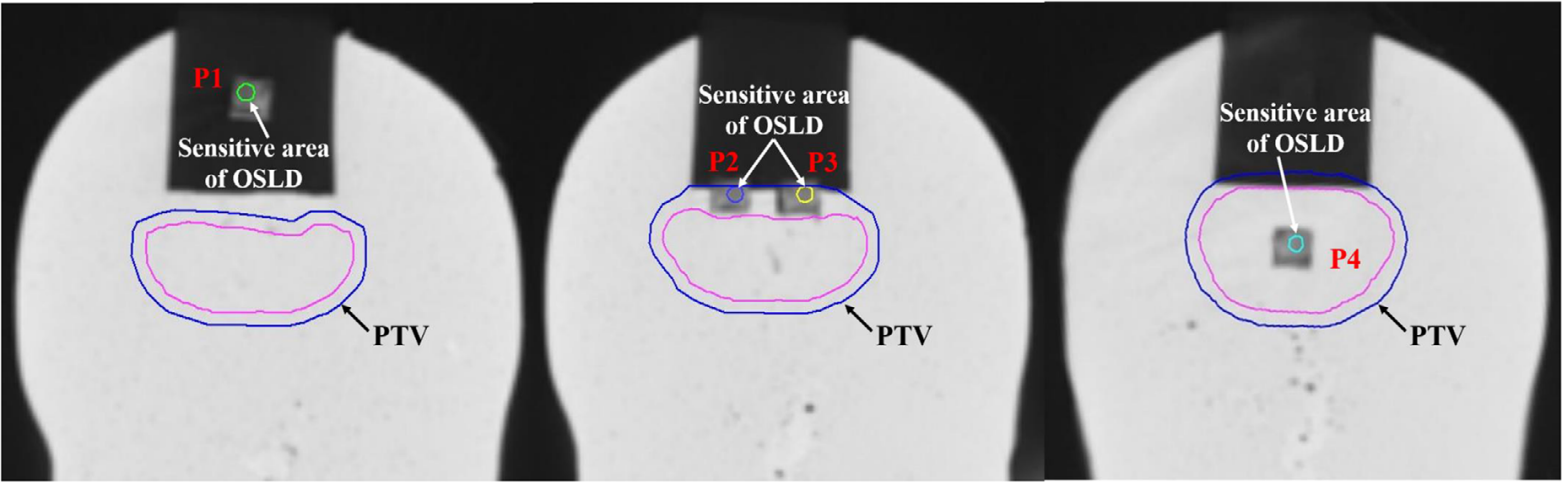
The space locations of four OSLDs and the PTV on CT images.
